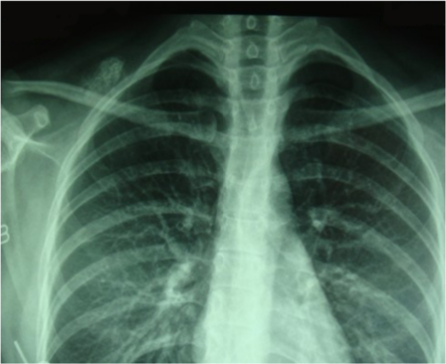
Chest X-ray demonstrating a mass with a high density shadow at the superior and posterior right clavicle
